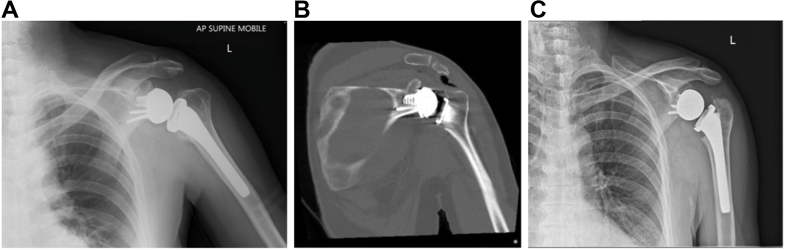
(A) Immediate postoperative radiographs, anteroposterior view, with stepoff between glenosphere and baseplate. (B) Post-operative CT, coronal view, demonstrating clear stepoff analogous to the radiograph. (C) 18-months postoperative radiograph with central screw backout and glenosphere dissociation demonstrated. *AP*, anteroposterior; *CT*, computed tomography.
